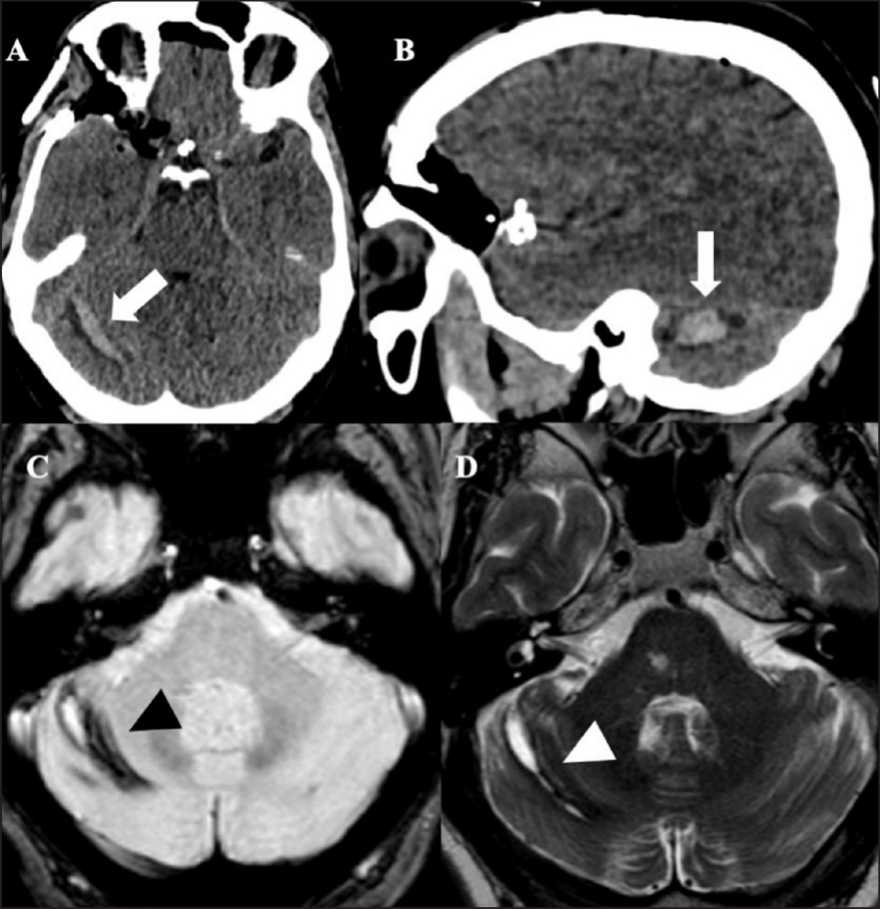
Patient 1. (A, B) Axial and sagittal CT images show a right cerebellar hemorrhage (*arrow*) exhibiting multiple stripes referred to as the ‘*zebra sign*’. (C) Axial T2 gradient-echo and (D) axial T2 weighted-images confirm hemosiderin deposits in the right cerebellar hemisphere (*arrowheads*).
